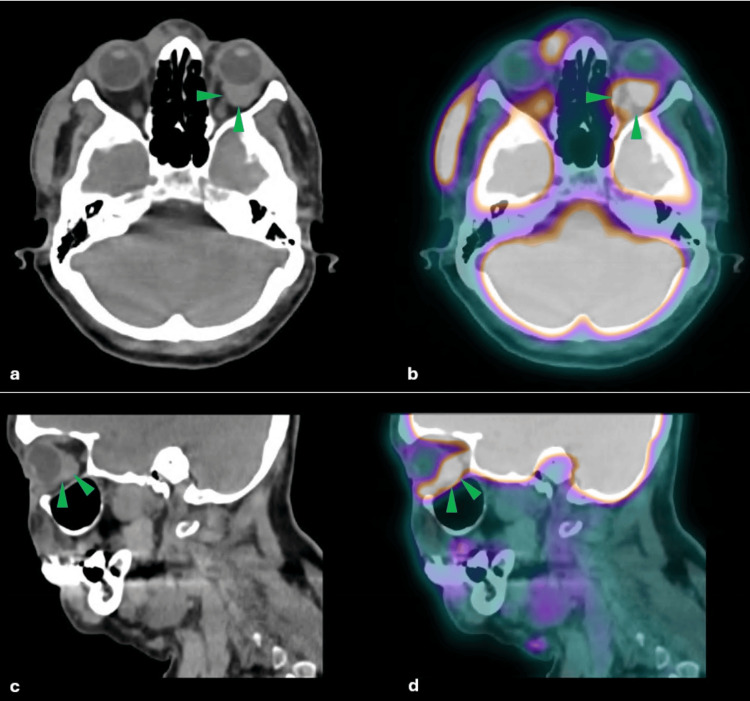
(a) Axial CT and (b) axial 18F-FDG PET/CT images demonstrating a hypermetabolic soft-tissue lesion within the left intraconal space (green arrowhead) in close contact with the posteroinferior aspect of the globe and partially encasing the optic nerve. (c) Sagittal CT and (d) sagittal 18F-FDG PET/CT images confirming the same lesion, measuring approximately 2.1 cm. 18F-FDG PET/CT: fluorine-18 fluorodeoxyglucose positron emission tomography/computed tomography.
